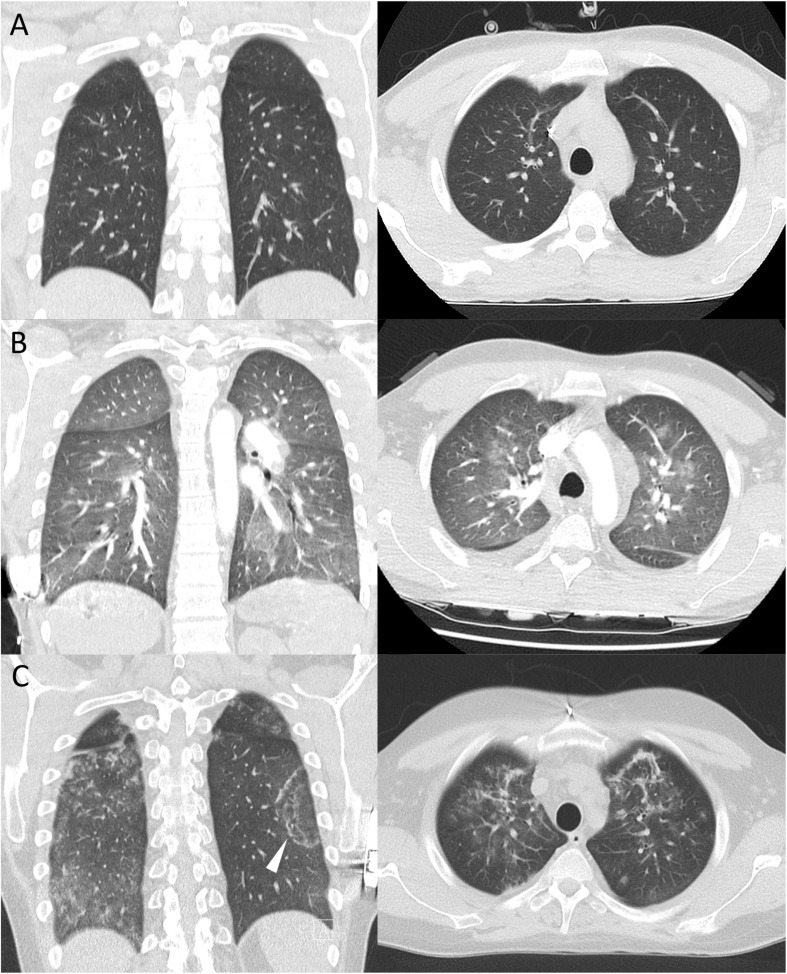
CT scans (coronal, axial) of the chest (A) after diagnosis of EGPA, unremarkable for pulmonary disease manifestation, (B) showing ground glass opacities and interlobular septal thickening after diagnosis of alveolar hemorrhage by bronchoalveolar lavage, (C) demonstrating bipulmonary ground glass opacities and consolidations with minor reticulation. Presence of reversed halo sign (arrow) as previously described in COVID-19 pneumonia.
